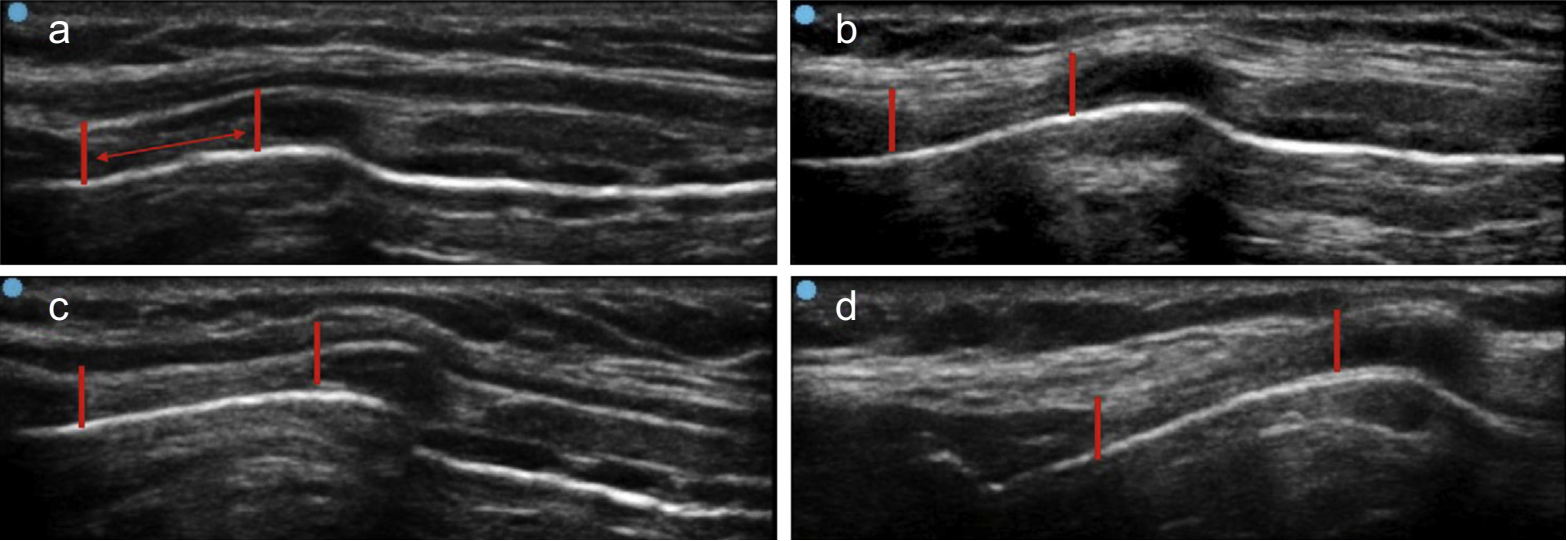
Four sequential front of neck longitudinal ultrasound images from a study participant showing change in CTM height. (a) Neutral neck position, no SAD; (b) extended neck position, no SAD; (c) neutral neck position, SAD *in situ*; (d) extended neck position, SAD *in situ*. Red markings indicate the superior and inferior extent of CTM. Note the cricoid cartilage pushed anteriorly. CTM, cricothyroid membrane; SAD, supraglottic airway device. (For interpretation of the references to colour in this figure legend, the reader is referred to the Web version of this article.)
